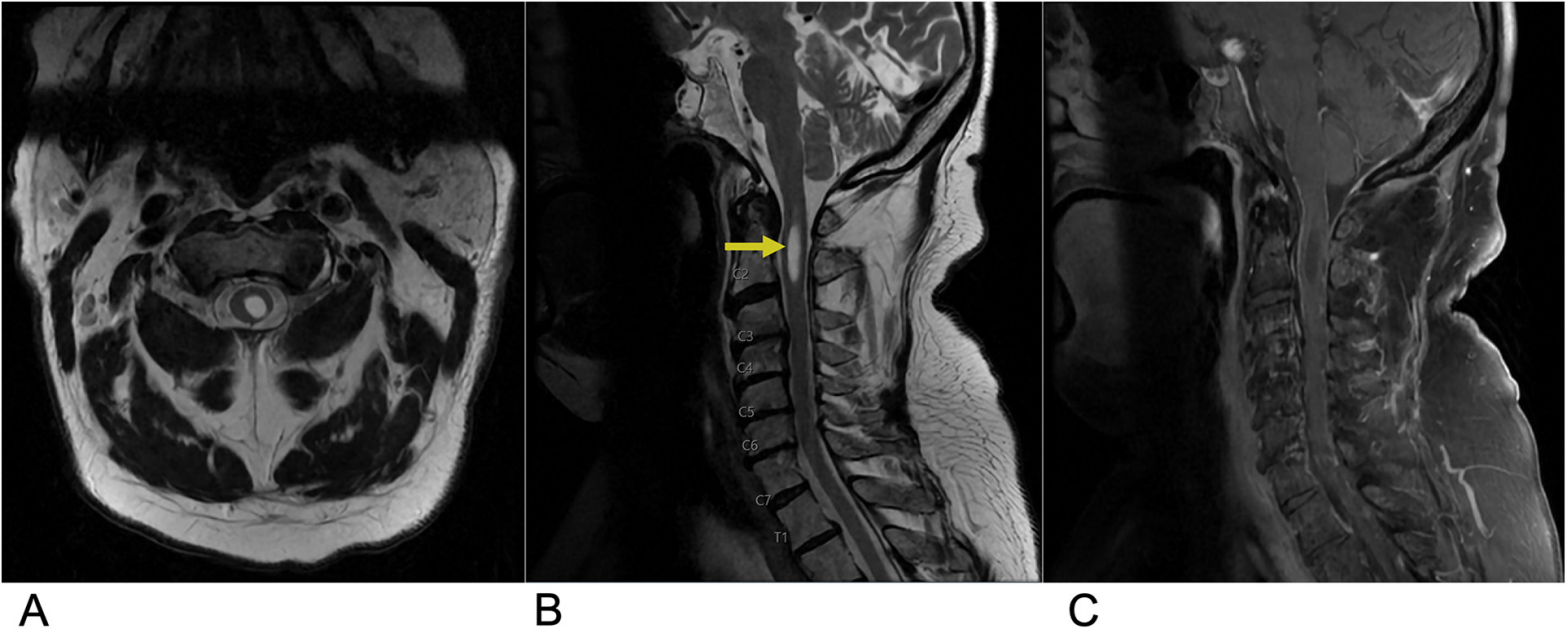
Hemangioblastoma. Axial T2 (A), sagittal T2 (B), & sagittal T1 FAT + C (C) MRI depicting a T2 hyperintense lesion (yellow arrow) at the C2 level representing a hemangioblastoma in this patient with a known history of Von Hippel Lindau Syndrome.
